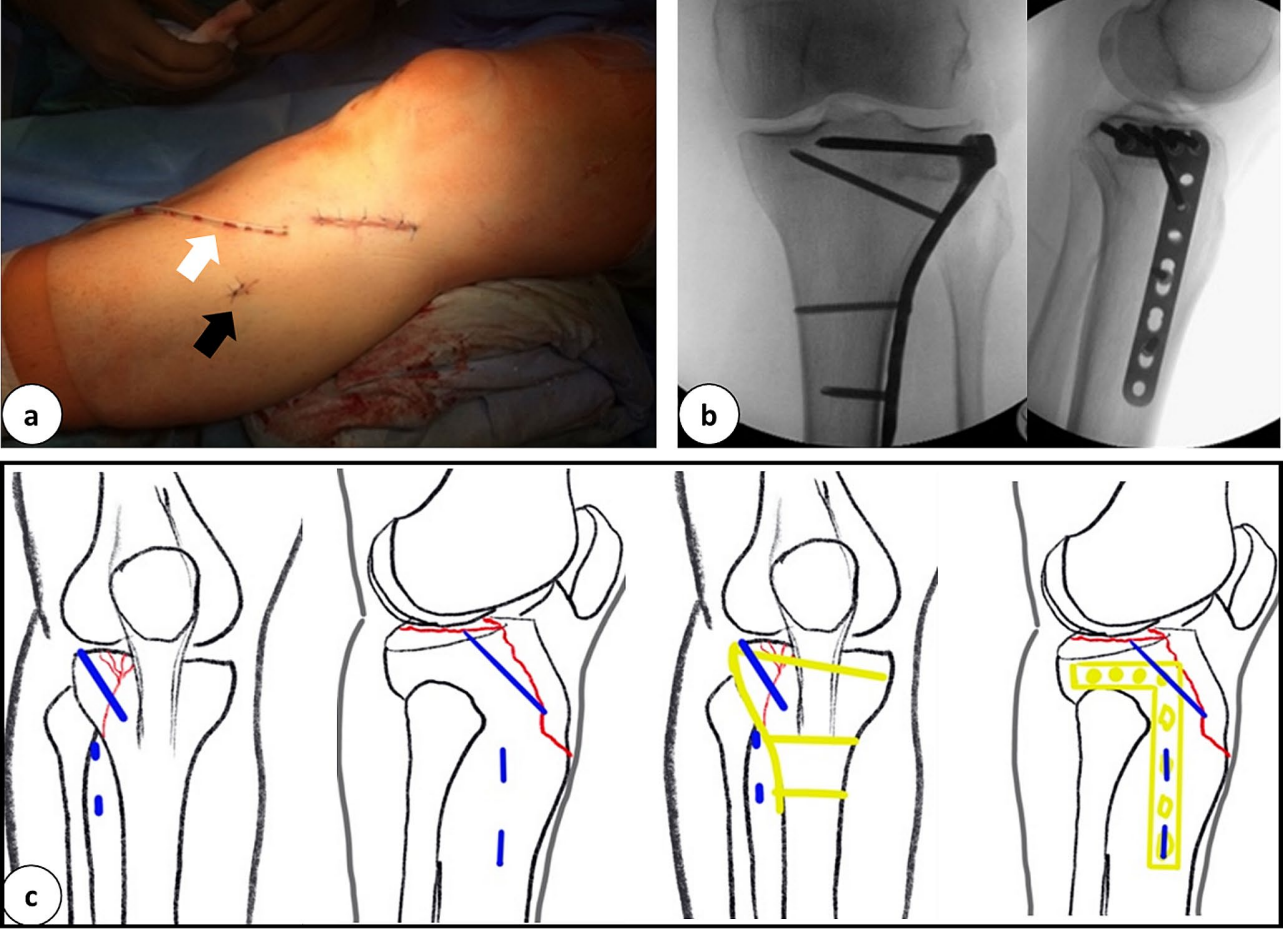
a: Posttraumatic X-Ray in 2 planes of a tibial shaft fracture (AO: 42C3). b: Post-operative X-Ray in 2 planes after performing a closed reduction and minimal invasive plate osteosynthesis. C: X-Ray in 2 planes 17 months post-surgical stabilization showing bony union
